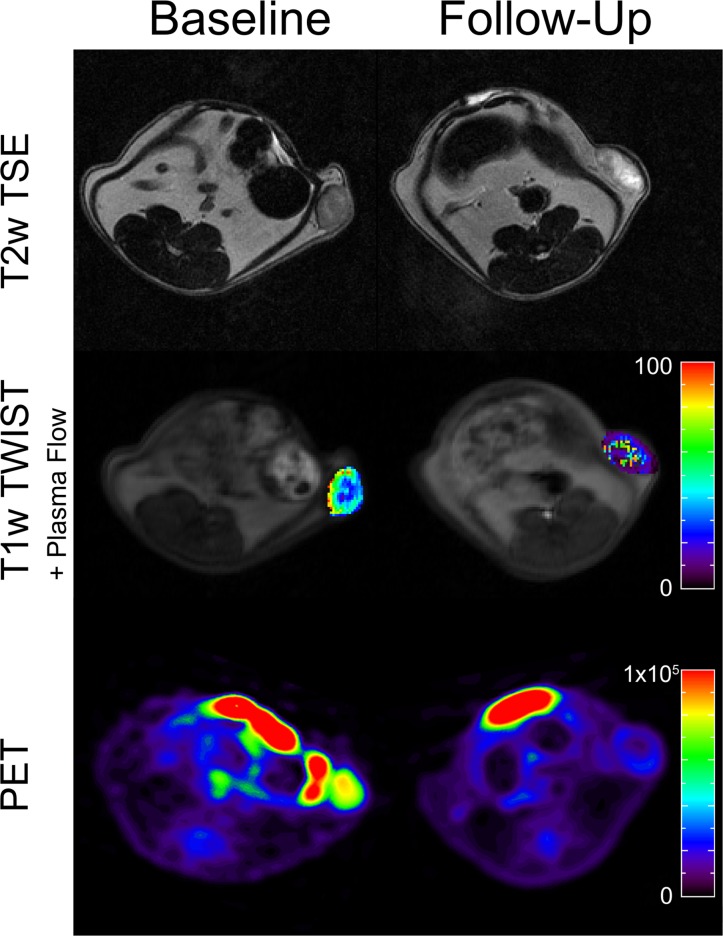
Representative axial images of the investigated human colon carcinoma xenografts including tumor morphology (T2w images, top row), tumor perfusion parameter maps (middle row) and ^18^F-FDG-PET (bottom row) before therapy (left column) and after a one-week treatment course of regorafenib (right column). Note the significant decrease of tumor plasma flow (mL/100mL/min) and tumor glucose metabolism (TTB) after regorafenib therapy between baseline and follow-up. The hyperintense central tumor regions on the T2-weighted image after therapy are consistent with increasing, therapy-induced tumor necrosis.
